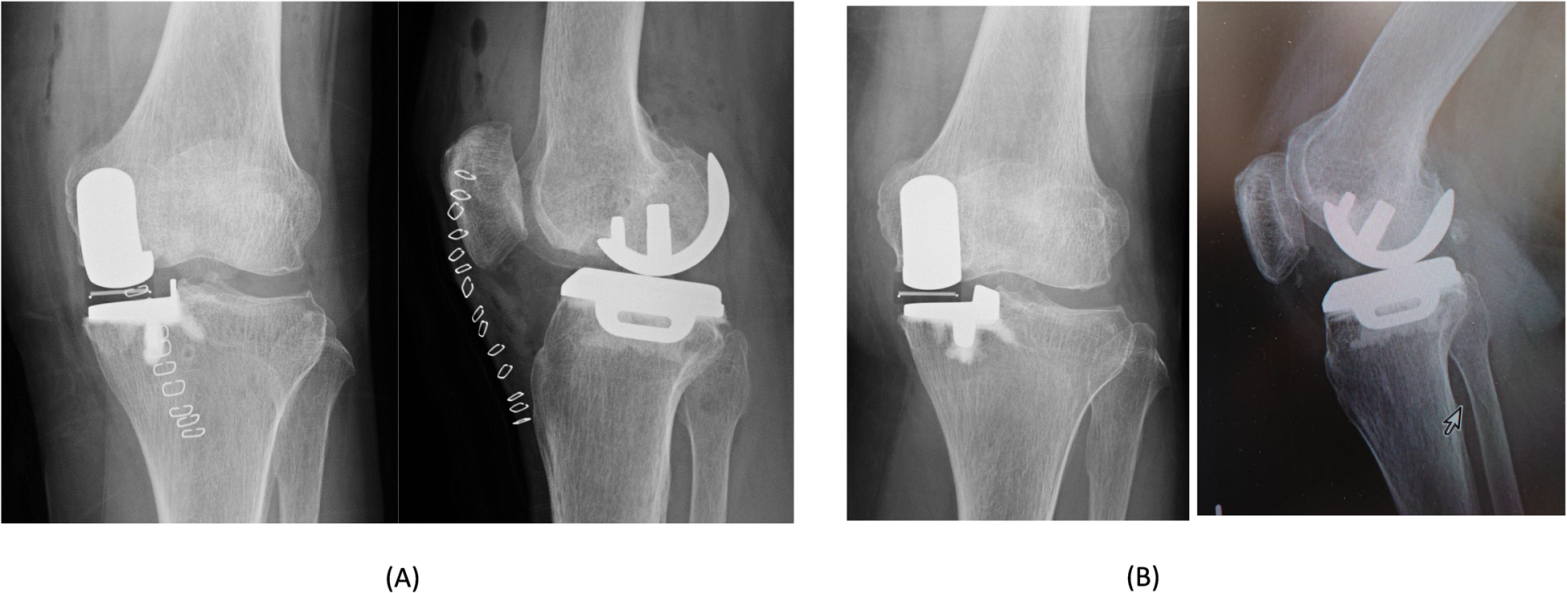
Case 1 radiographs. Postoperative plain radiographs of the left knee. a Postoperative radiographs at 1 year showing the migration of the femoral component (b)
